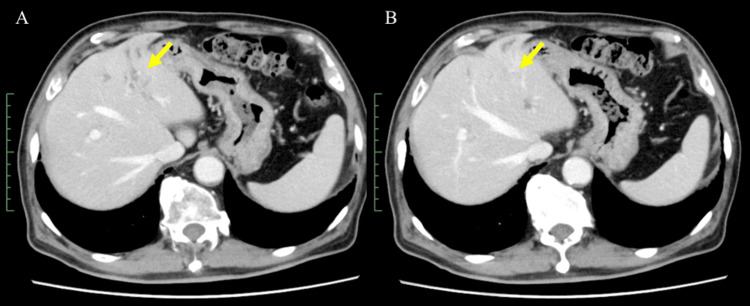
Abdominal computed tomography (CT) images obtained after treatment with pembrolizumab (A, B) Abdominal CT images showed significant shrinkage of the primary lesion (arrow).
